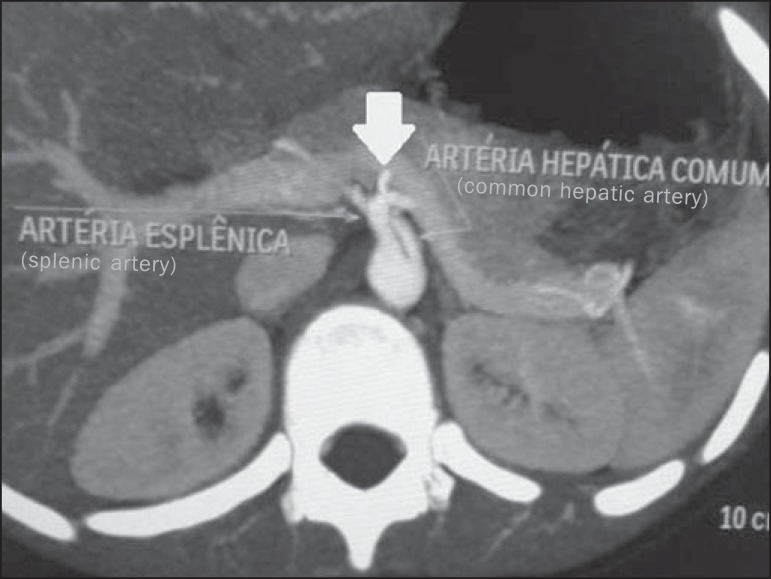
Contrast-enhanced axial computed tomography showing a splenogastic trunk
